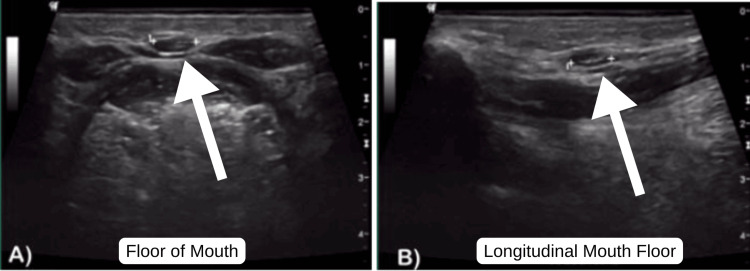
Cervical ultrasound showing an anechoic lesion with regular borders and a thin wall at the infrahyoid level. Transverse (A) and longitudinal (B) views are shown.
